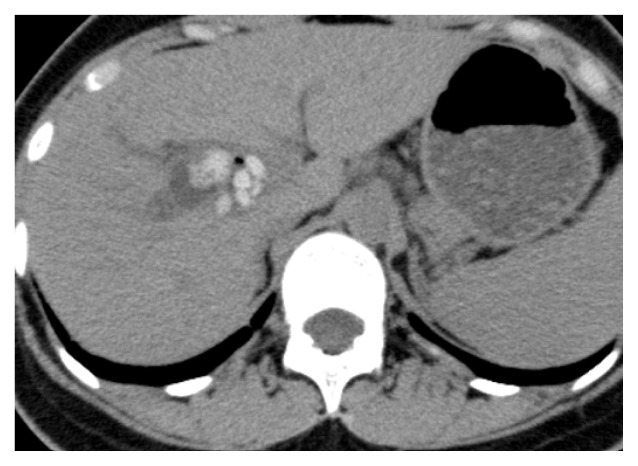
Multiple intrahepatic stones in the right hepatic duct were detected by CT scan.
